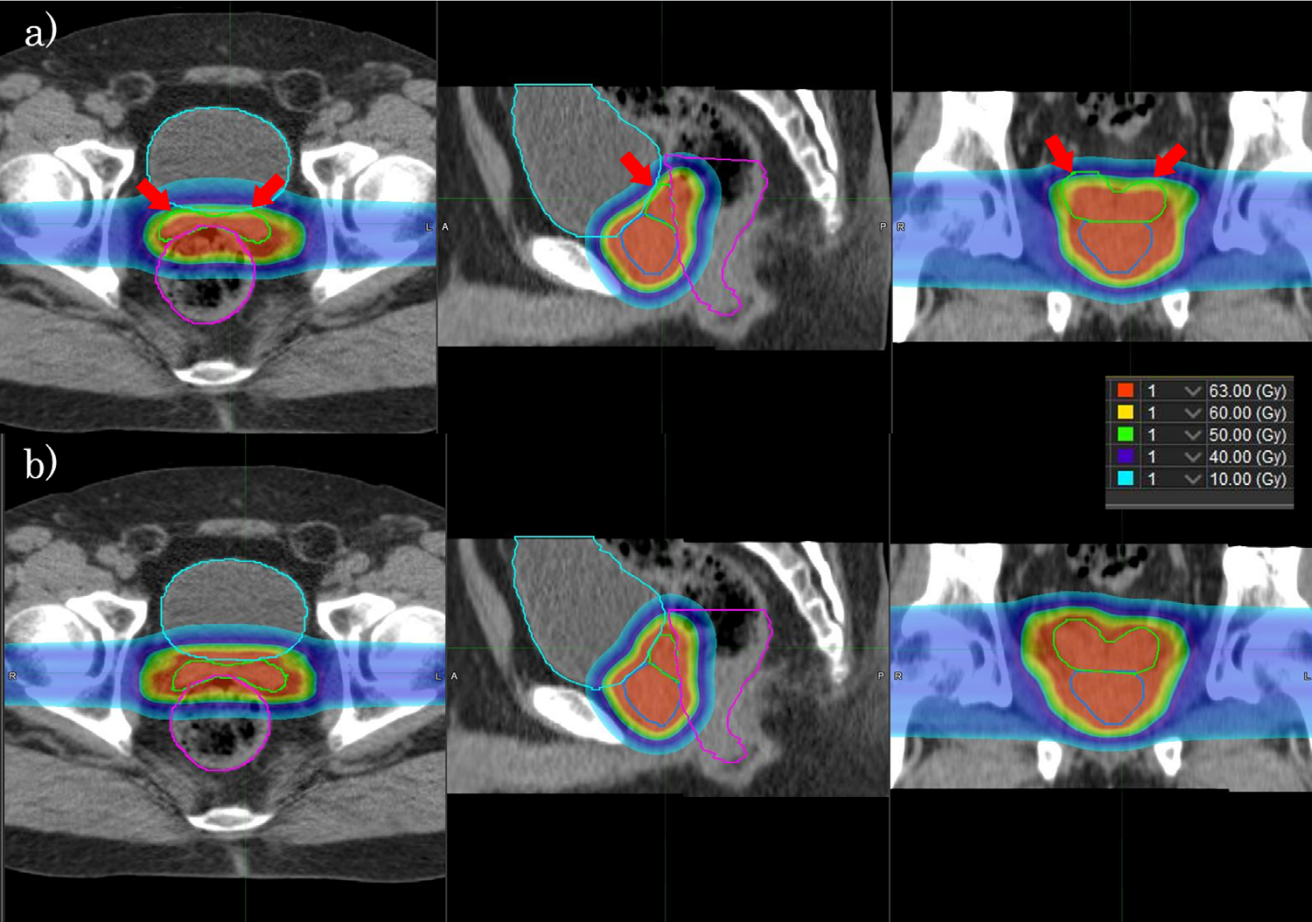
Representative example of the estimated actual dose distributions of non‐adaptive radiotherapy (NART) (a) and daily adaptive radiotherapy (DART) (b) using the same daily computed tomography (CT) images in the axial, sagittal, and coronal planes. The regions of interest (ROI) are displayed in the prostate (blue), seminal vesicle (SV) (light green), rectum (magenta), and bladder (light blue). The notable difference between two treatment strategies is failing to cover the anterior cranial side of SV in NART
